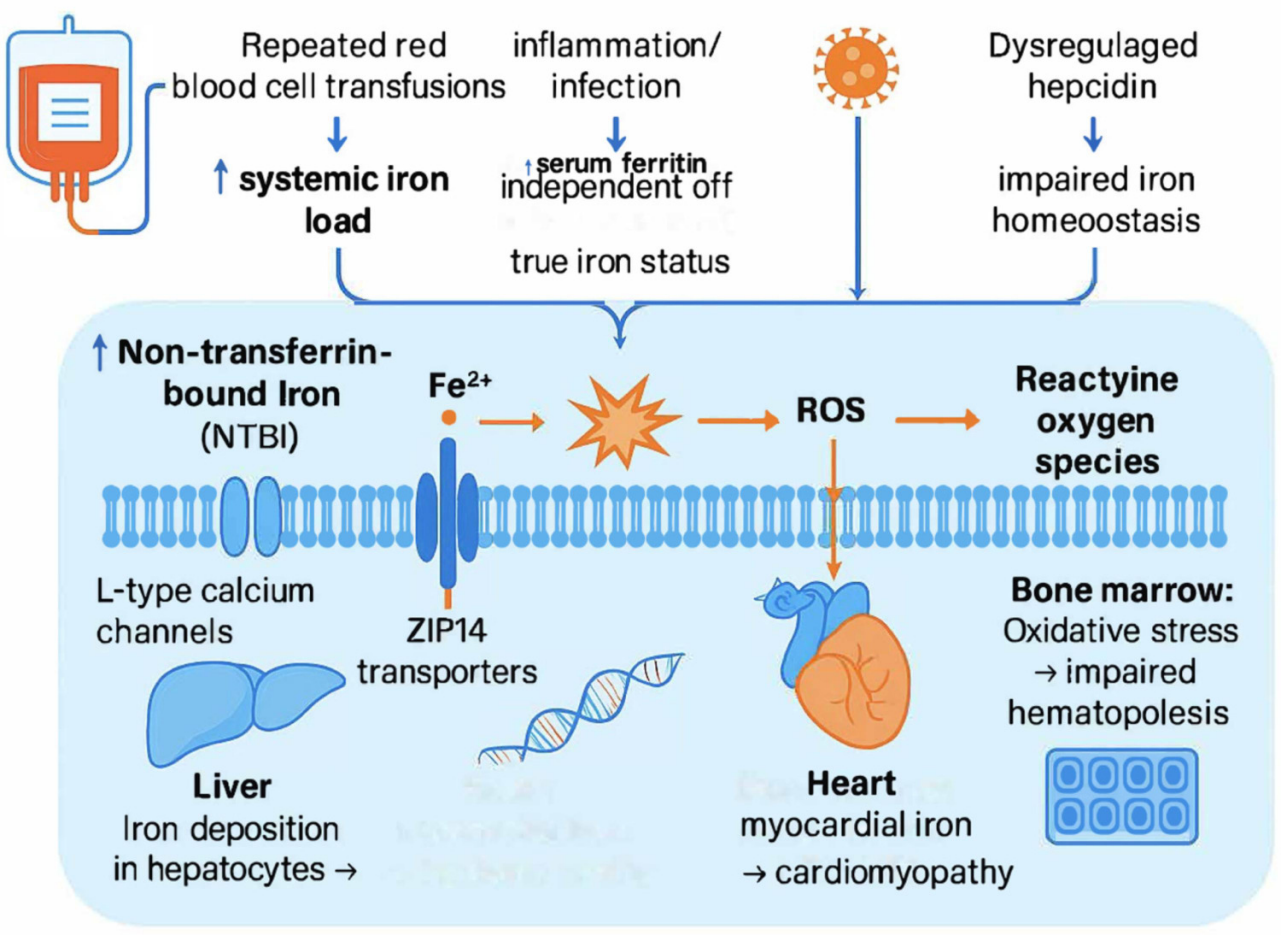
Mechanistic overview of transfusional iron overload in pediatric cancer patients. Repeated blood transfusions and inflammation increase systemic iron load and serum ferritin levels, leading to the accumulation of non–transferrin-bound iron (NTBI). Excess NTBI enters cells through L-type calcium channels and ZIP14 transporters, catalyzing the production of reactive oxygen species (ROS). These reactive molecules cause oxidative stress, resulting in hepatic and myocardial iron deposition and impaired hematopoiesis in the bone marrow. Early detection through combined serum ferritin and T2* MRI monitoring can prevent irreversible organ damage.
